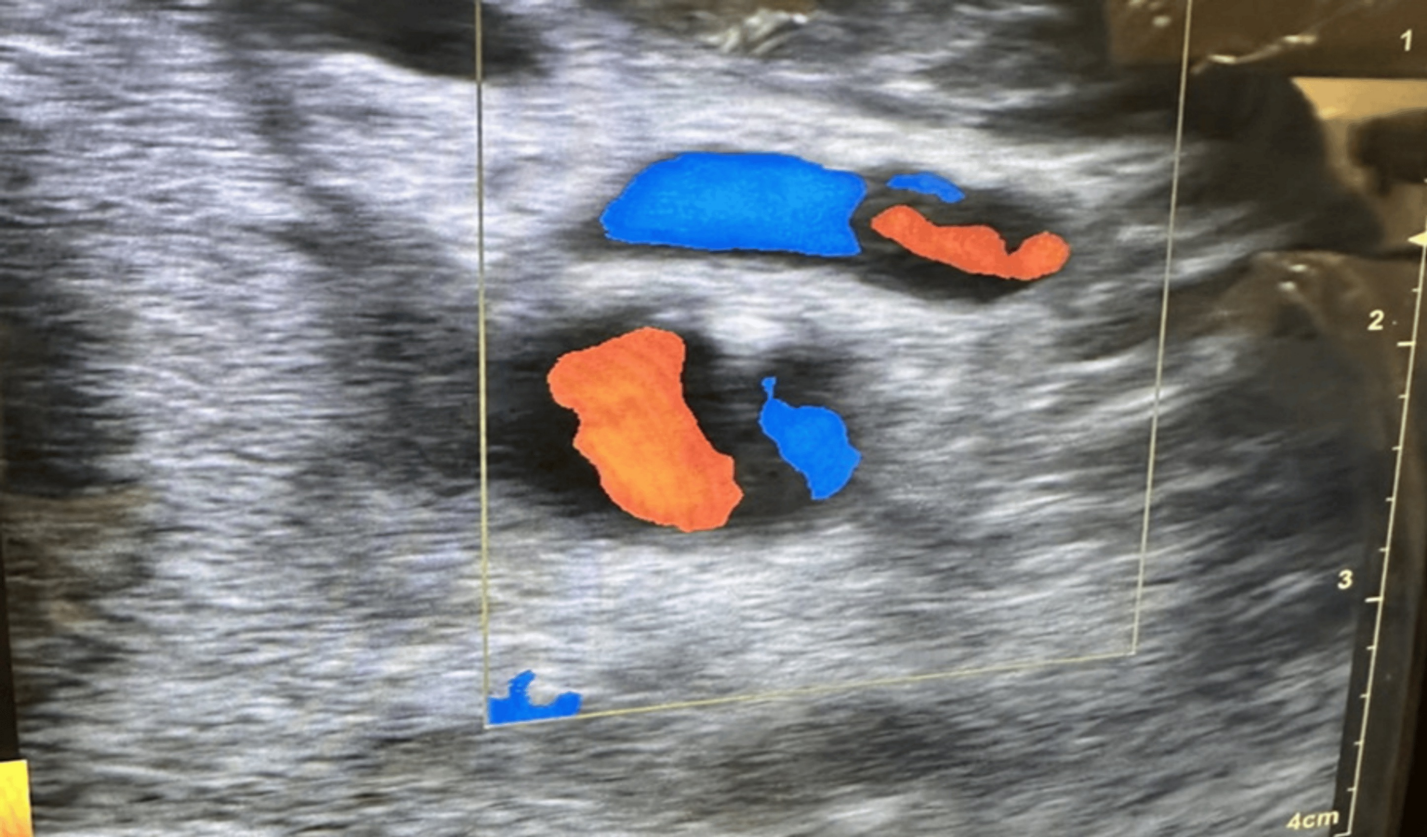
Bedside ultrasound image following arteriotomy closure with the Perclose ProGlide suture device.
